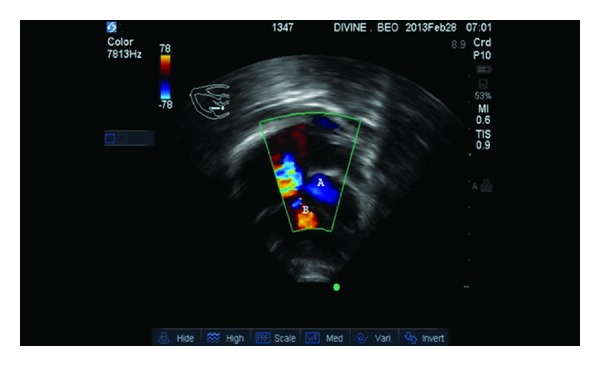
Color flow Doppler from an apical four-chamber view showing systolic flow from LV to RA though the adherent septal and anterior tricuspid leaflets. Note the Gerbode jet (A) and the VSD jet (B).
